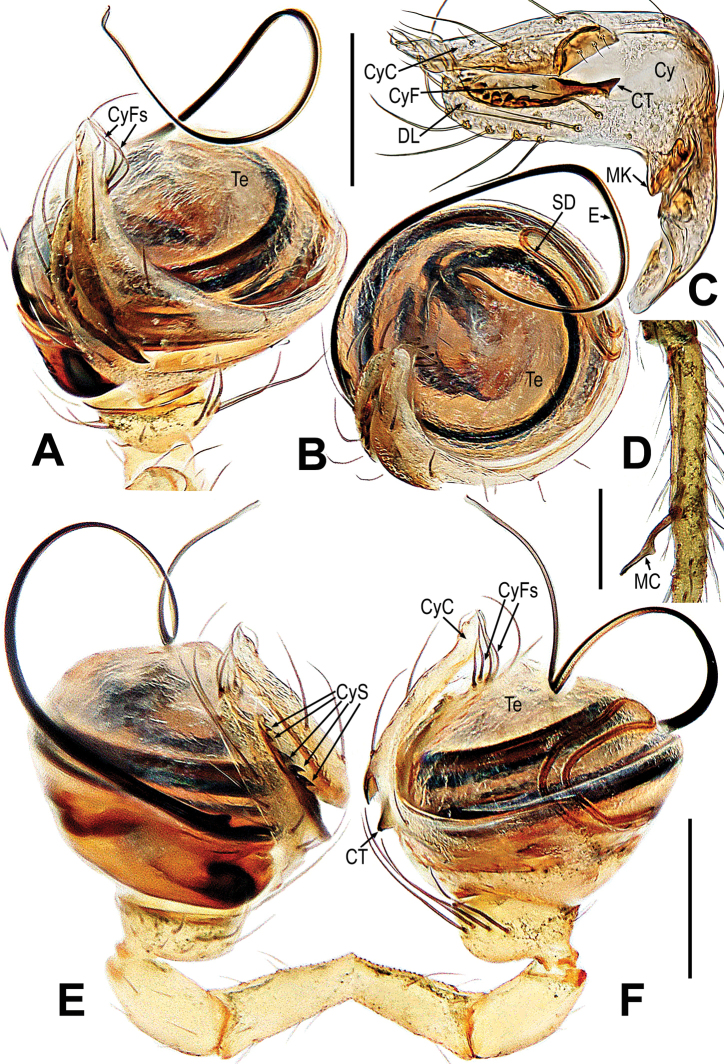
Mosuheguomu* sp. nov. A, B, E, F male palp C cymbium D male left metatarsus I A, C ventral B apical D, E prolateral F retrolateral. Abbreviations: CT = cymbial tooth; Cy = cymbium; CyC = cymbial conductor; CyF = cymbial fold; CyFs = setae on cymbial fold; CyS = cymbial serrula; DL = distal lobe on cymbium; E = embolus; MC = Metatarsal clasping spine; MK = median keel on cymbium; SD = spermatic duct; Te = tegulum; Ti = palpal tibia. Scale bars: 0.20 mm (A–C, E, F); 0.10 mm (D).
